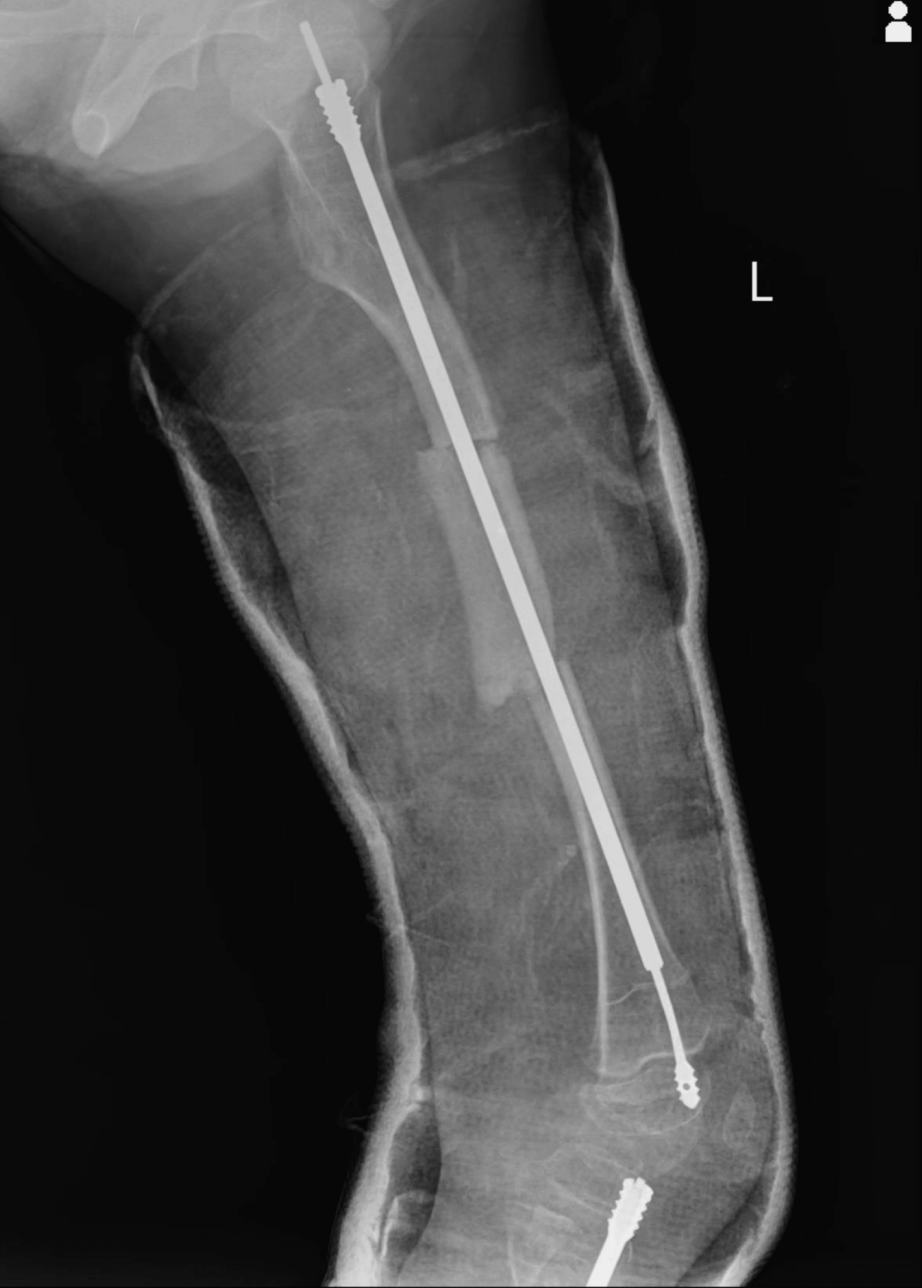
Follow-up X-ray of the patient's femur after rod implantation.
